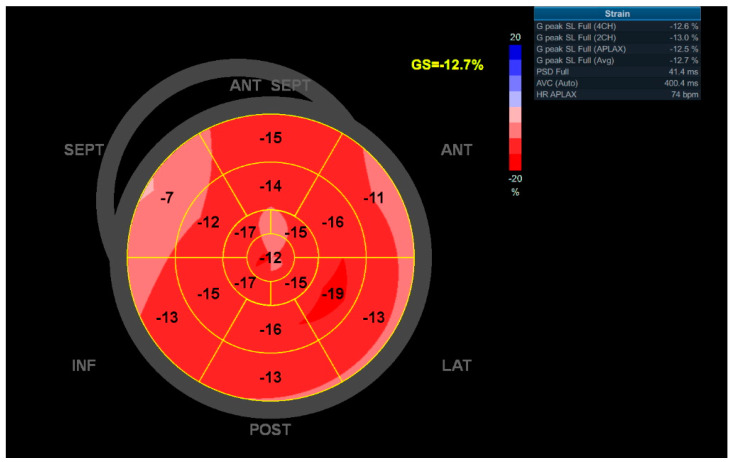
Figure 2 shows a Bulls-Eye longitudinal strain map of a 36-year-old woman who was diagnosed with CHL in 1999 and treated with chemotherapy and mantle field radiotherapy. Echocardiography at index visit in 2015 showed impaired LV GLS of −12.7% and LVEF of 48%. This patient had a grade 1 diastolic dysfunction and an E/e’ mean of 8.8. In 2017, this patient was admitted for HF due to non-ischemic cardiomyopathy, most probably caused by prior chemotherapy.
